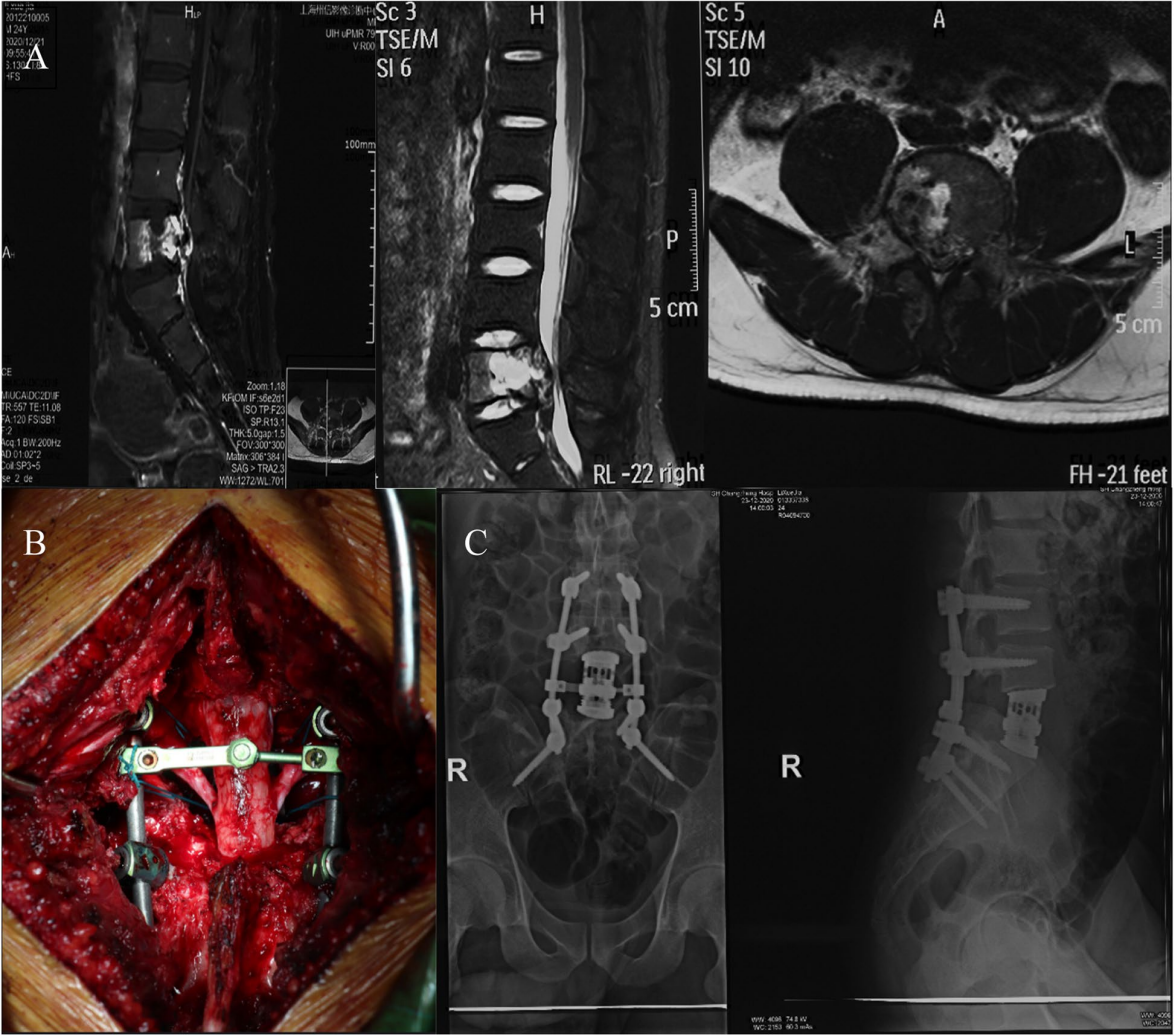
Images of a 24-year-old man with L5 metastasis from liver cancer who received TES. A T1-, contrast-enhanced T2-weighted and T2-MR images show the vertebral tumor extending into the spinal canal with dural sac compression. B Intraoperative photographs show the TES performed. C Postoperative X-ray
